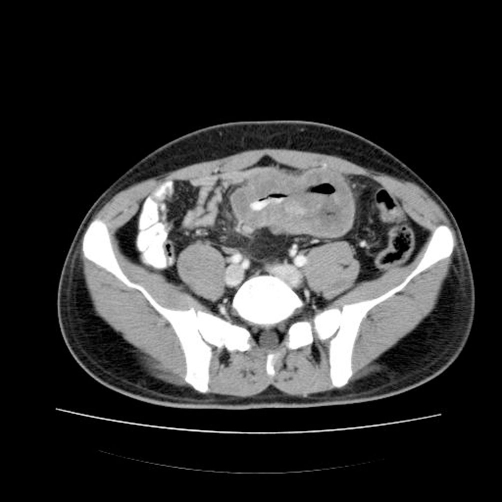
CT image showing some thickened ileal loops and an increased in volume mesenteric lymph node.
